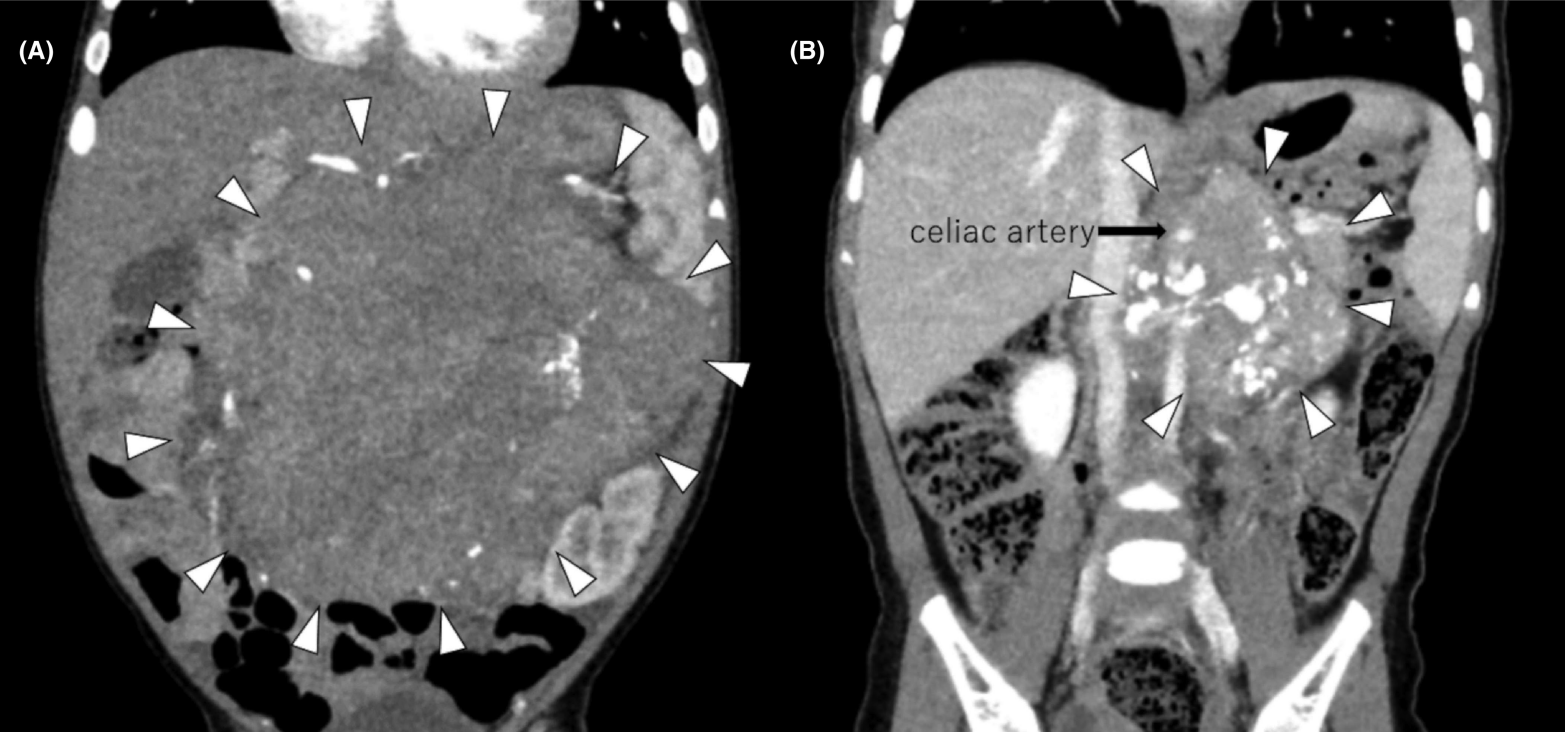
Chemotherapy was administered, and a CT scan showed the tumor (arrowhead) had shrunk with residual celiac artery involvement (arrow). (A) Before chemotherapy. (B) After the ninth course of chemotherapy. CT, computed tomography.
